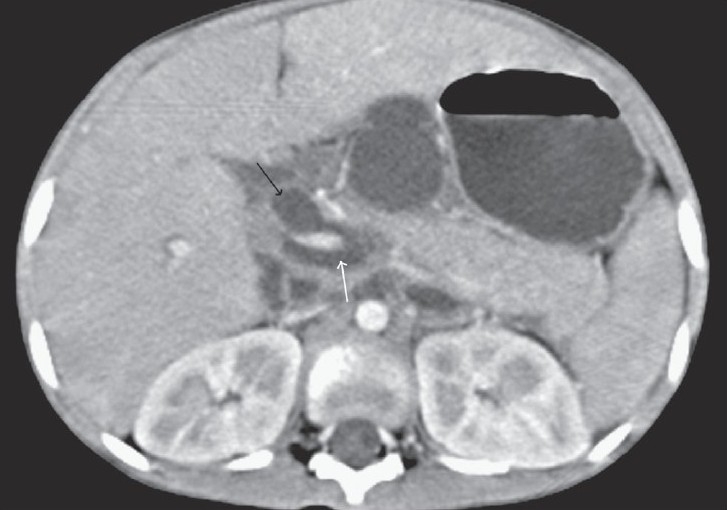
Transaxial contrast-enhanced CT scan shows a dilated suprapancreatic CBD (black arrow) communicating with a retroperitoneal collection (white arrow) that is surrounding the portal venous confluence
